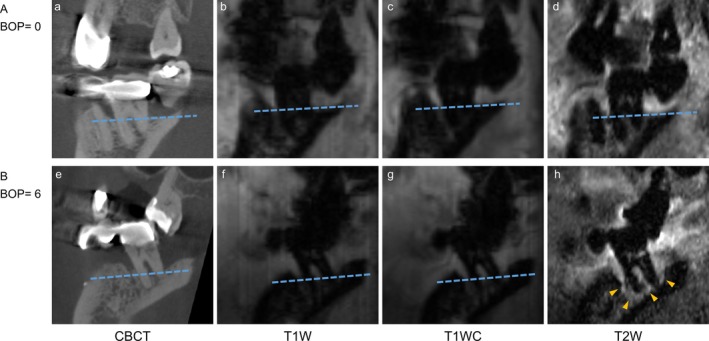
Images of teeth 36 and 37 with zero bleeding sites of a 61‐year‐old female patient (A) and tooth 47 with six bleeding sites of a 51‐year‐old male patient (B). Around teeth with no to minimal bleeding, periodontal lesions are expressed similarly across modalities, slightly larger in dMRI than in CBCT (dashed blue line) (A). The increasing severity of bleeding is expressed modestly and similarly in CBCT, T1W and T1WC, but evidently on T2W (B). T2W is showing an additional area with T2W‐only signal alteration beyond the bone loss (yellow arrowhead). Imaging modalities include cone‐beam computed tomography (CBCT: a, e), T1‐weighted (T1W: b, f), contrast‐enhanced T1‐weighted (T1WC: c, g) and T2‐weighted (T2W: d, h).
